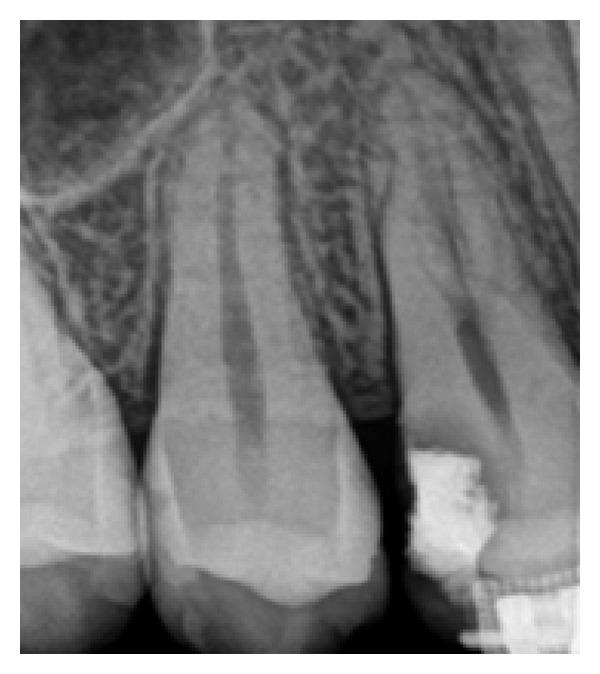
Initial periapical radiography. Radiopacity alteration in the middle third of the pulp chamber of tooth 14, suggesting trifurcation of the root canal.
